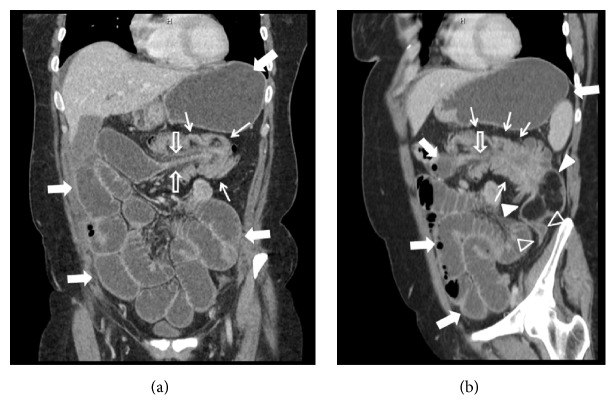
Contrast-enhanced CT, portal venous phase, multiplanar reconstructions (coronal plane, (a), and paracoronal plane, (b)). The same features described in (a) can be appreciated: the dilated stomach and small bowel loops (full arrows), the right colon, intussusceptum (empty arrows), the left colon, intussuscipiens (thin arrows), the colonic lipoma (full arrowheads), and the collapsed left colon distal to the lipoma (empty arrowheads).
